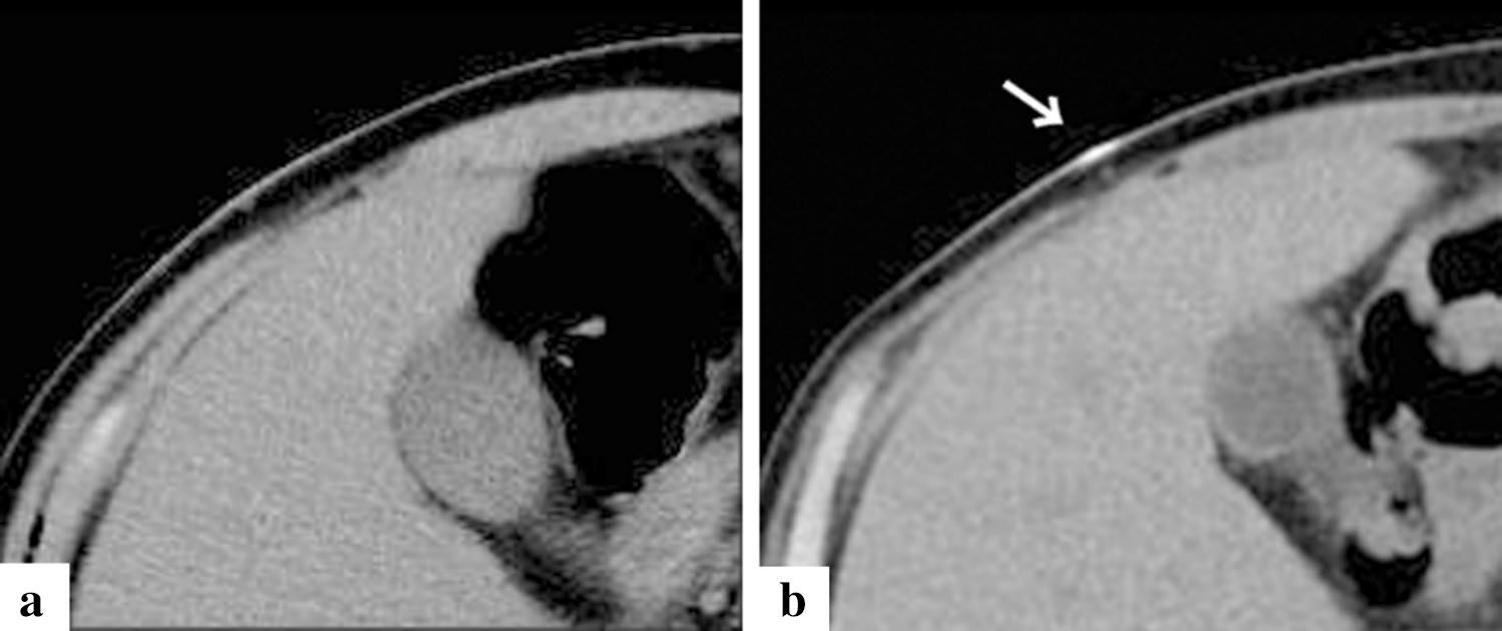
Representative case of dermal calcification in a 50-year-old patient (case no. 2). Computed tomography (CT) imaging on day 41 since the onset of symptoms (a) and after one month of the admission (b). CT imaging on admission showed no abnormalities in the skin (a), and CT imaging one month after admission showed calcification in the skin (b, white arrow)
